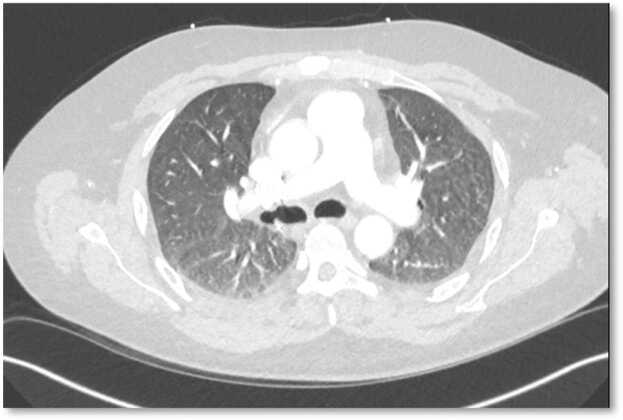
Chest CT axial image with demonstration of the diffuse bilateral ground glass opacities.
